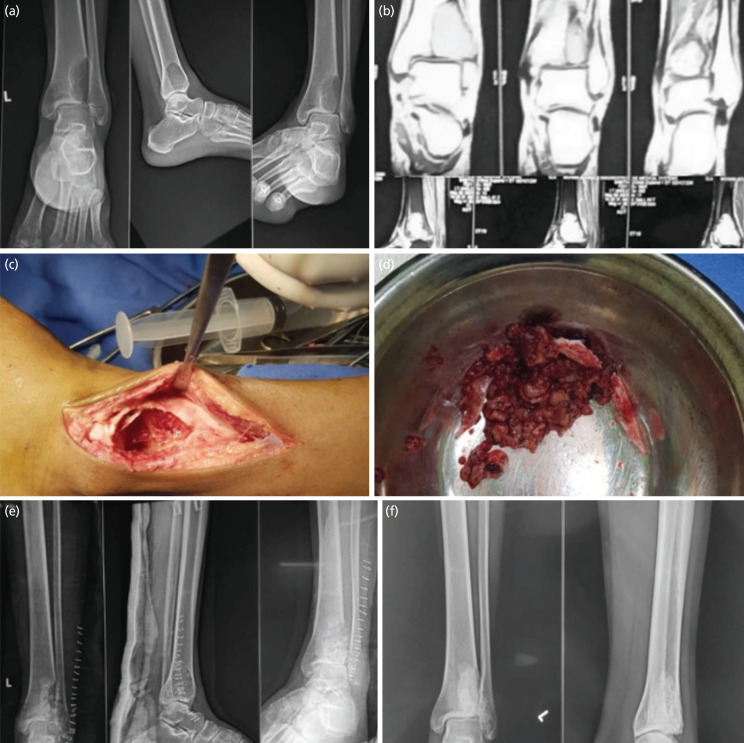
(a) Radiograph of the involved ankle showing a lytic lesion at the tibiofibular syndesmotic interface extending into the subarticular region of the distal tibia. (b) Magnetic Resonance Imaging of the involved ankle confirming the diagnosis of GCT. (c) Intra-operative image after proper curettage and chemical treatment of the tumour. (d) Intra-operative picture of the curetted tumour tissue (e)post operative radiograph after cementation of the defect. (f) One year post-operative radiograph.
